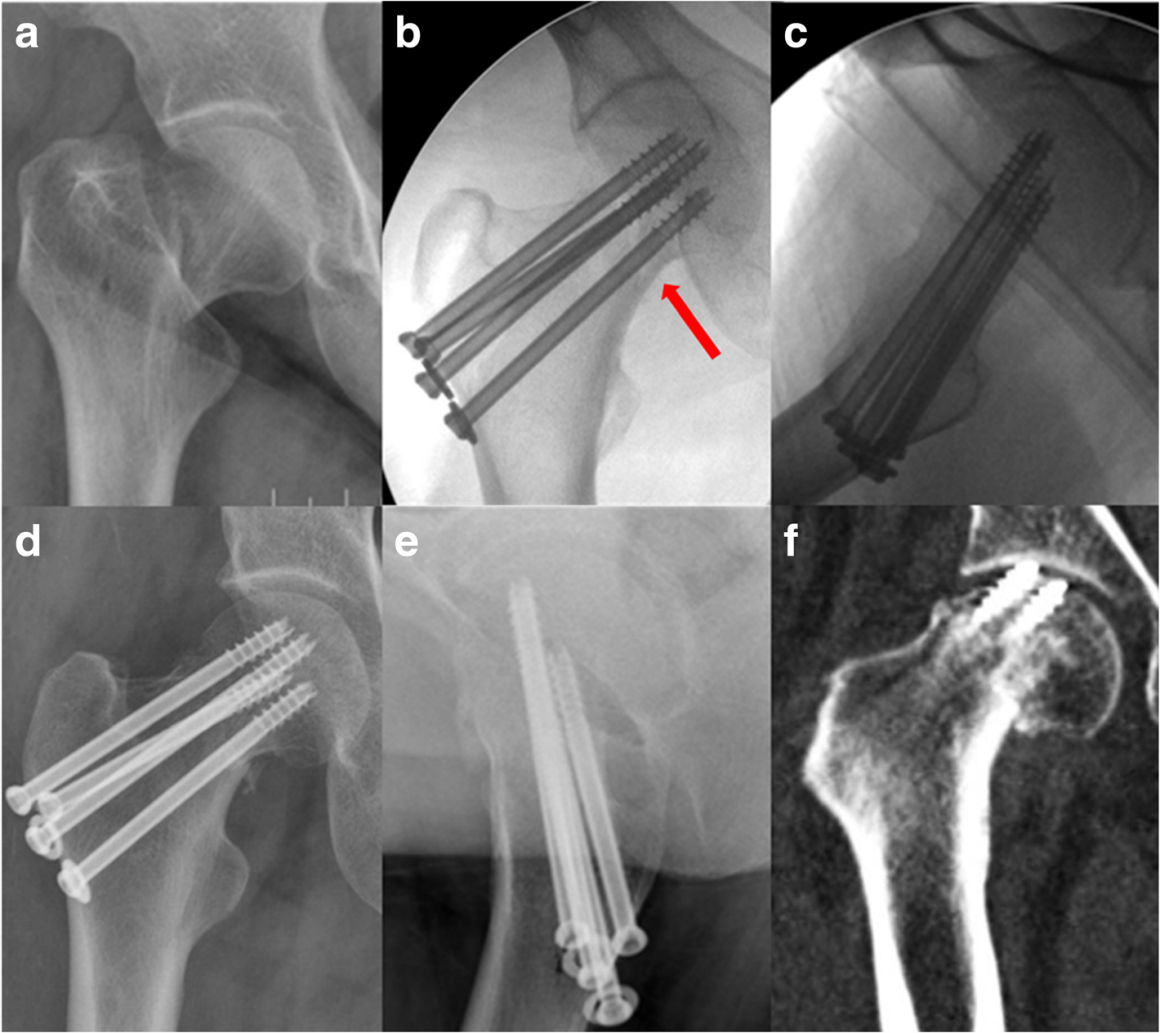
AP view of a displaced femoral neck fracture in a 55-year-old female (a). The fracture obtained smooth anatomic reduction (red arrow) according to intraoperative fluoroscopies (b, c), and the fracture changed into slightly varus (≤ 10°) 3 days postoperatively (d, e). CT showed the fracture displaced to varus (> 10°) 4 months postoperatively, which resulted in early failure as the screws cut out from the femoral head (f)
